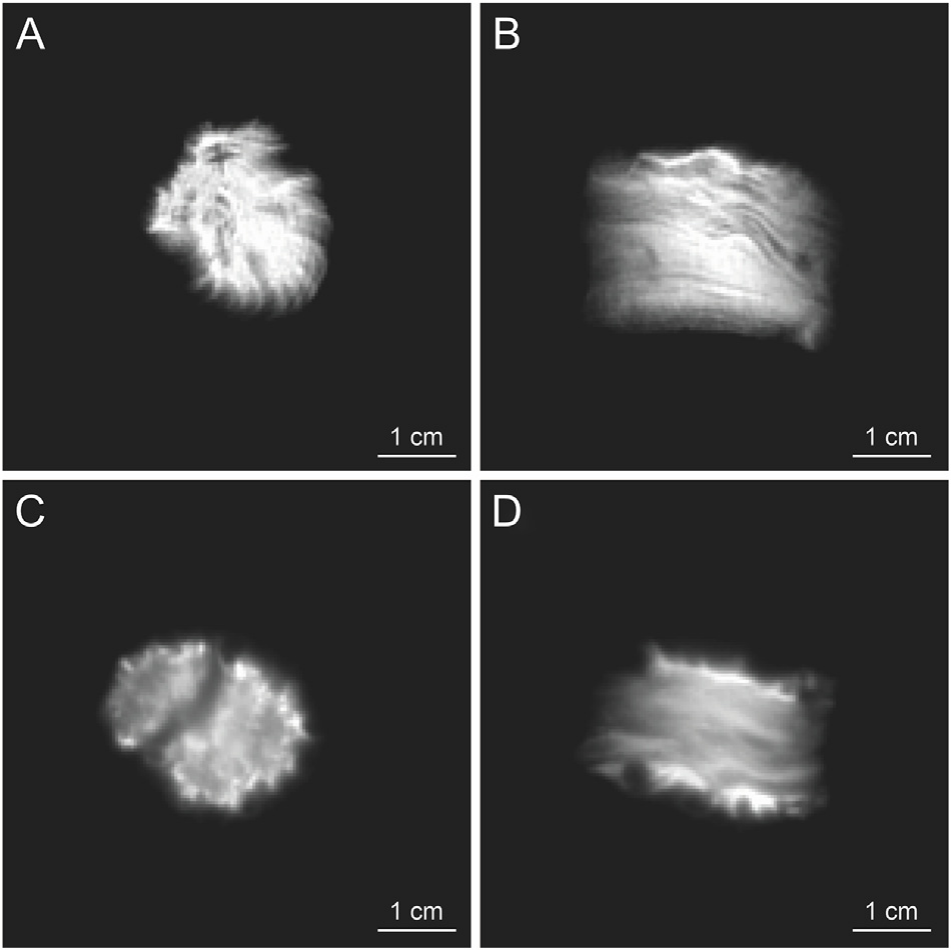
Differences of *Salvia miltiorrhiza* during sweating processing revealed by magnetic resonance imaging (MRI). (A, B) Axial (A) and sagittal (B) images of the root before sweating processing. (C, D) Axial (C) and sagittal (D) images of the root after sweating processing.
